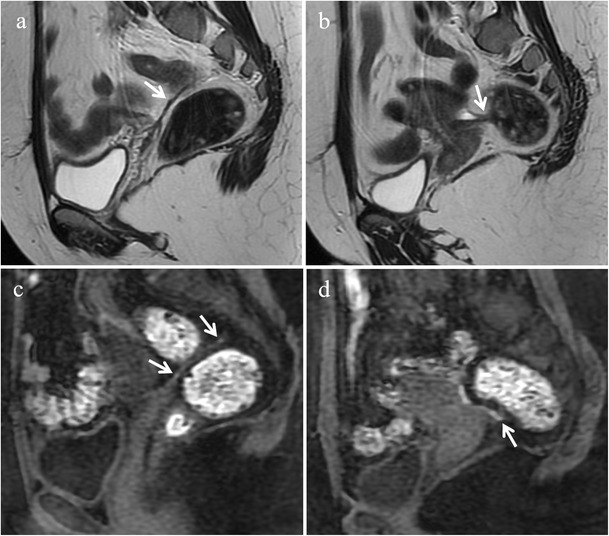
Endometriosis of the uterosacral ligaments in a 35-year-old woman with multiple DIE lesions. The same patient as in Fig. 10. (a, b) Sagittal T2-weighted images show hypointense thickening of both uterosacral ligaments (white arrows). (c, d) Sagittal fat-suppressed T1-weighted images demonstrate hyperintense spots within the ligaments (white arrows), representing hemorrhagic foci
